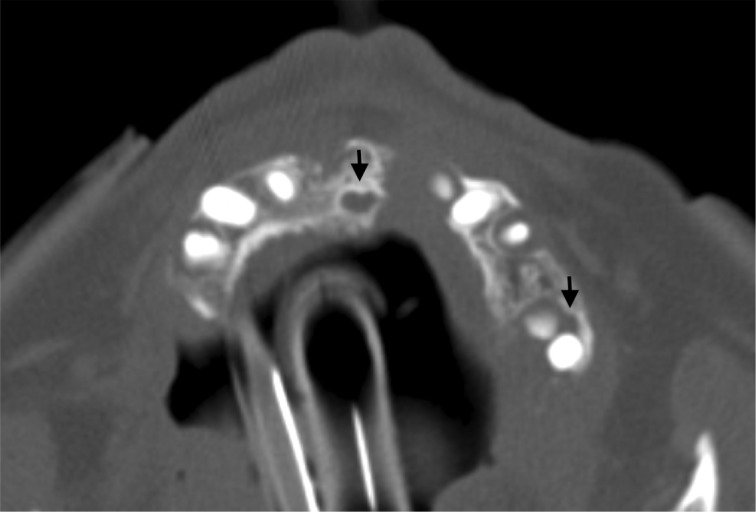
Computed tomography maxillofacial imaging demonstrating large periapical abscesses adjacent to the maxillary teeth.
